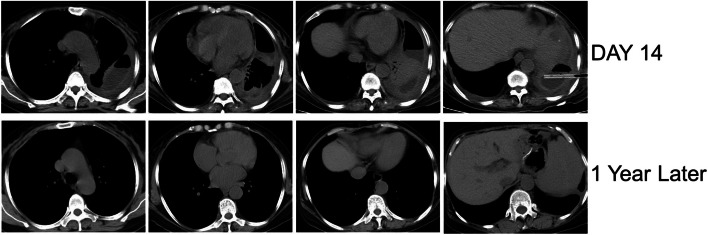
Show the chest CT scans of the patient on the 14th day of hospitalization and 1 year later, showing the left lung was gradually re-expanded, the left pleural effusion was gradually reduced, and the left pleural thickening was gradually decreased
